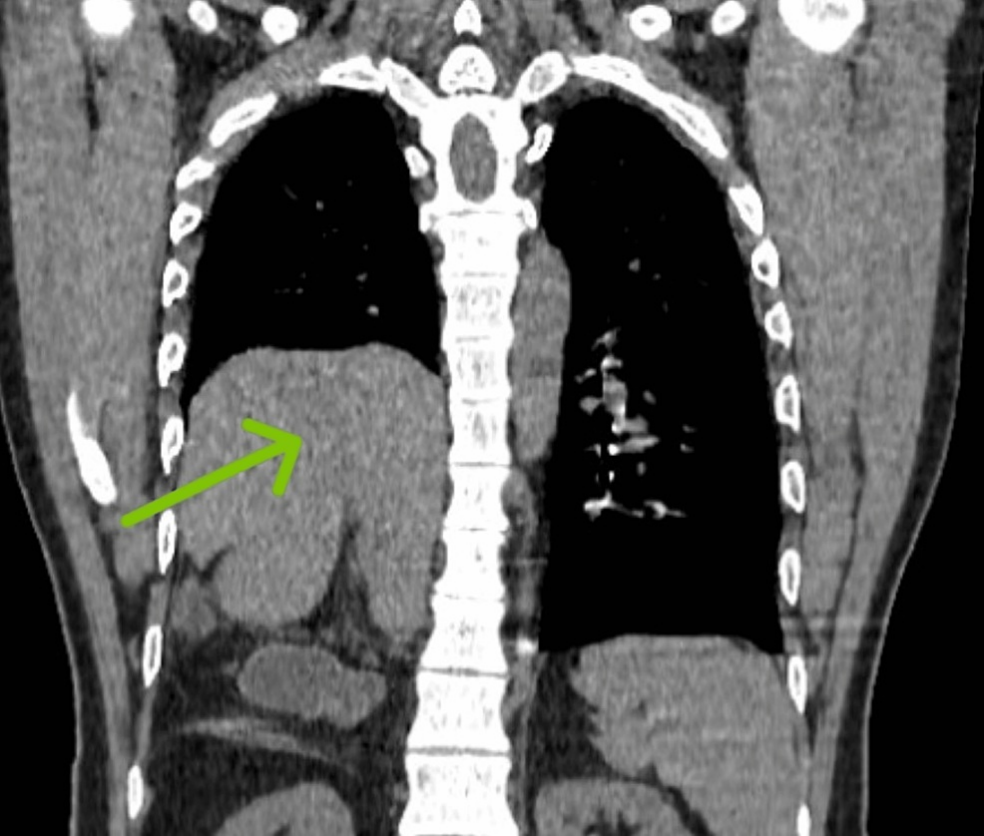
Coronal section of the CT scan of the chest showing herniation of the liver into the thorax (green arrow)
